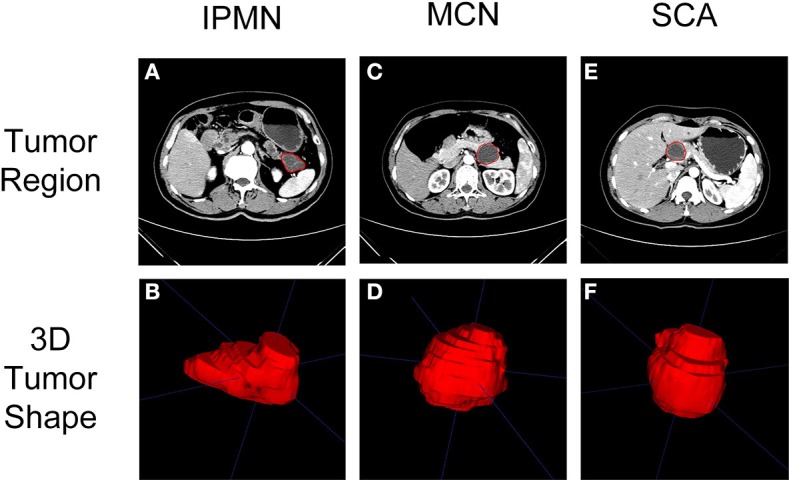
Typical CT imaging (arterial phase) of SCA, MCN, and IPMN were shown in (A,C,E). The region of interest on CT imaging after delineation were shown in (B,D,F).
